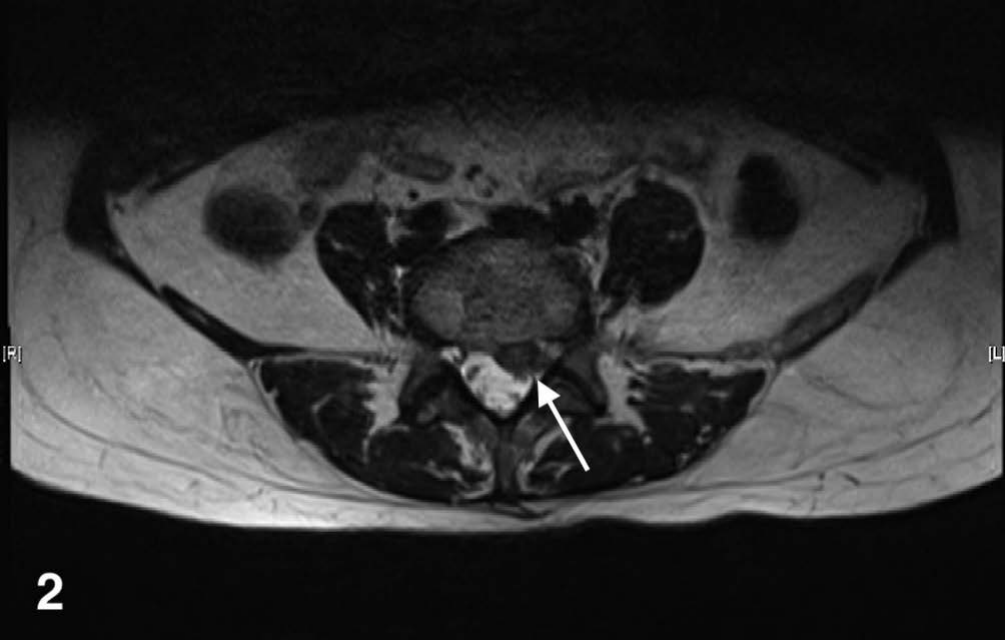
MRI horizontal plane of lumbar spine before UBE surgery. MRI = magnetic resonance imaging.
